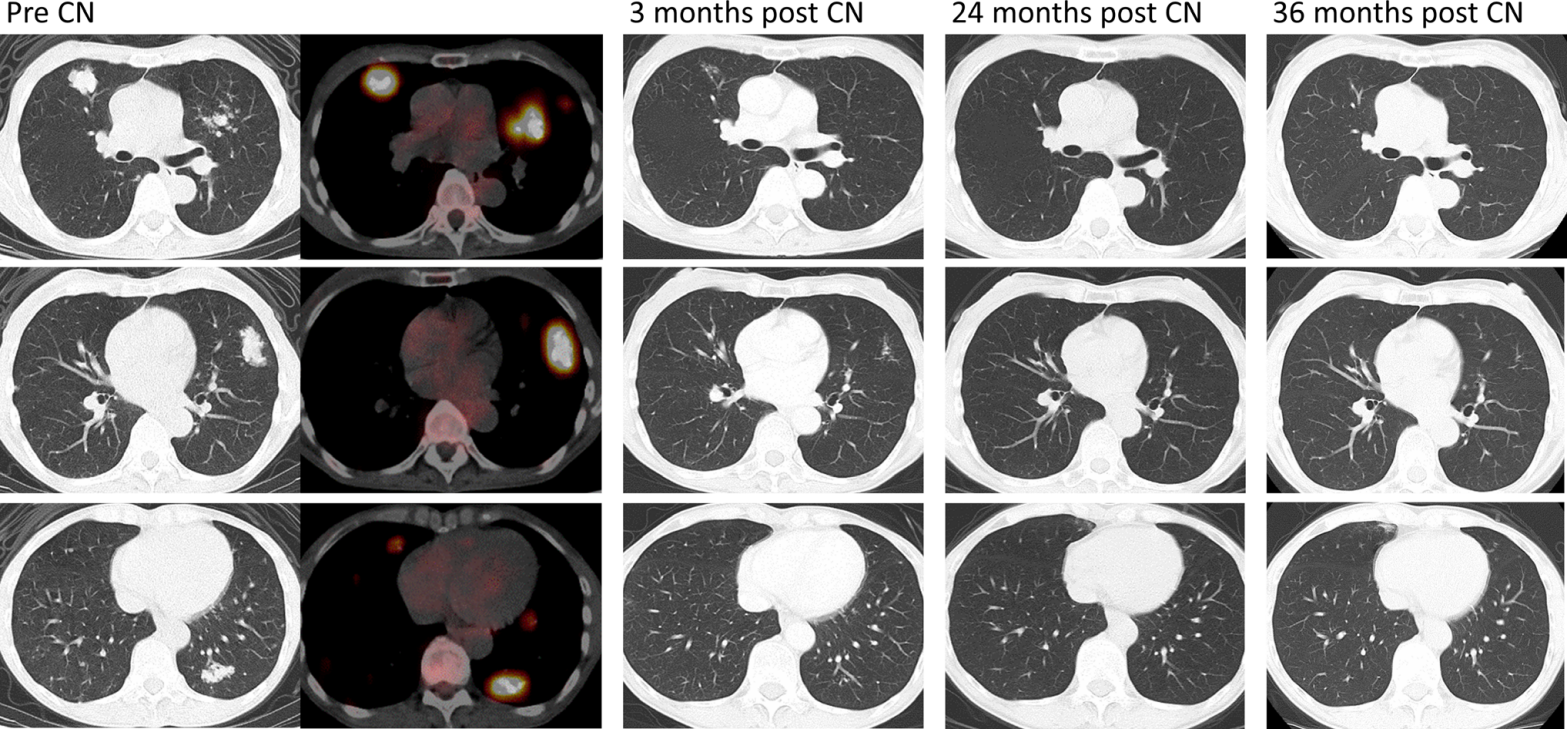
Chest CT scan findings following CN, showing the spontaneous complete regression of multiple pulmonary metastases.
